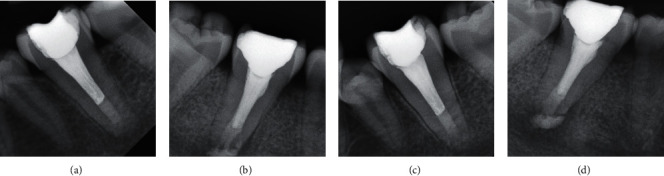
(a, b) Postobturation radiograph #36 and #46. (c, d) One-year follow-up radiograph #36 and #46.
